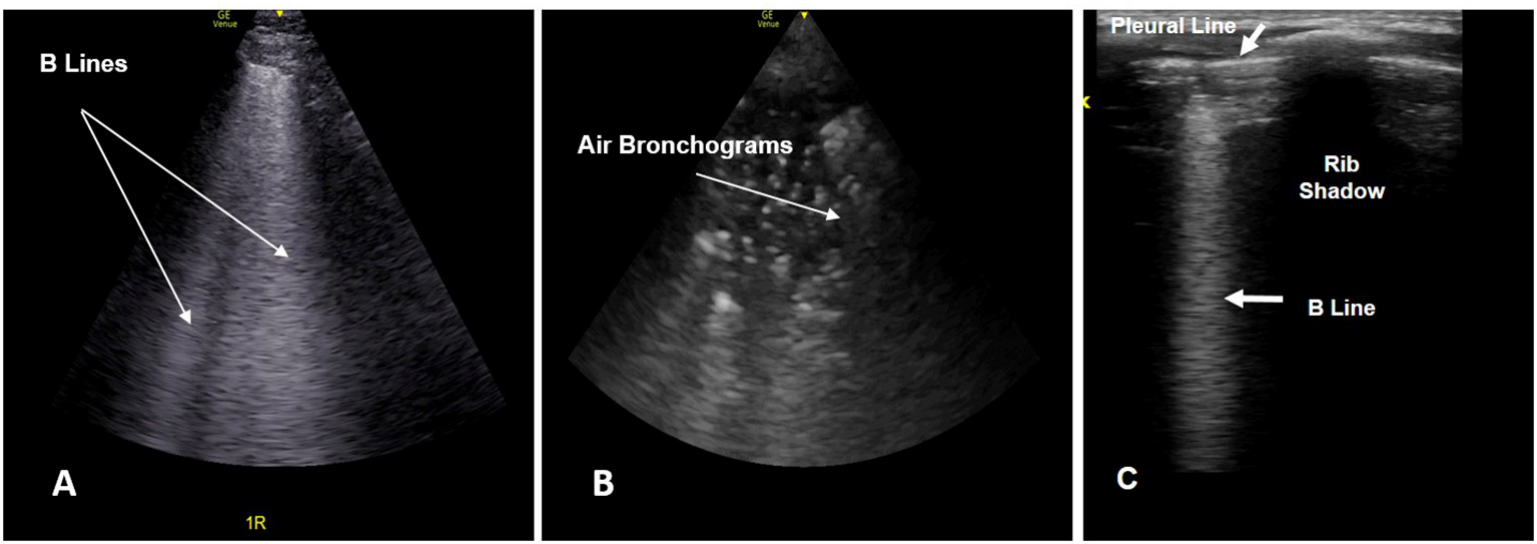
(A) Lung ultrasound image showing multiple B lines (arrow). (B) Lung ultrasound image showing air bronchograms (arrow) giving a “speckled” appearance. (C) Lung ultrasound image obtained using a linear probe showing a single B line.
